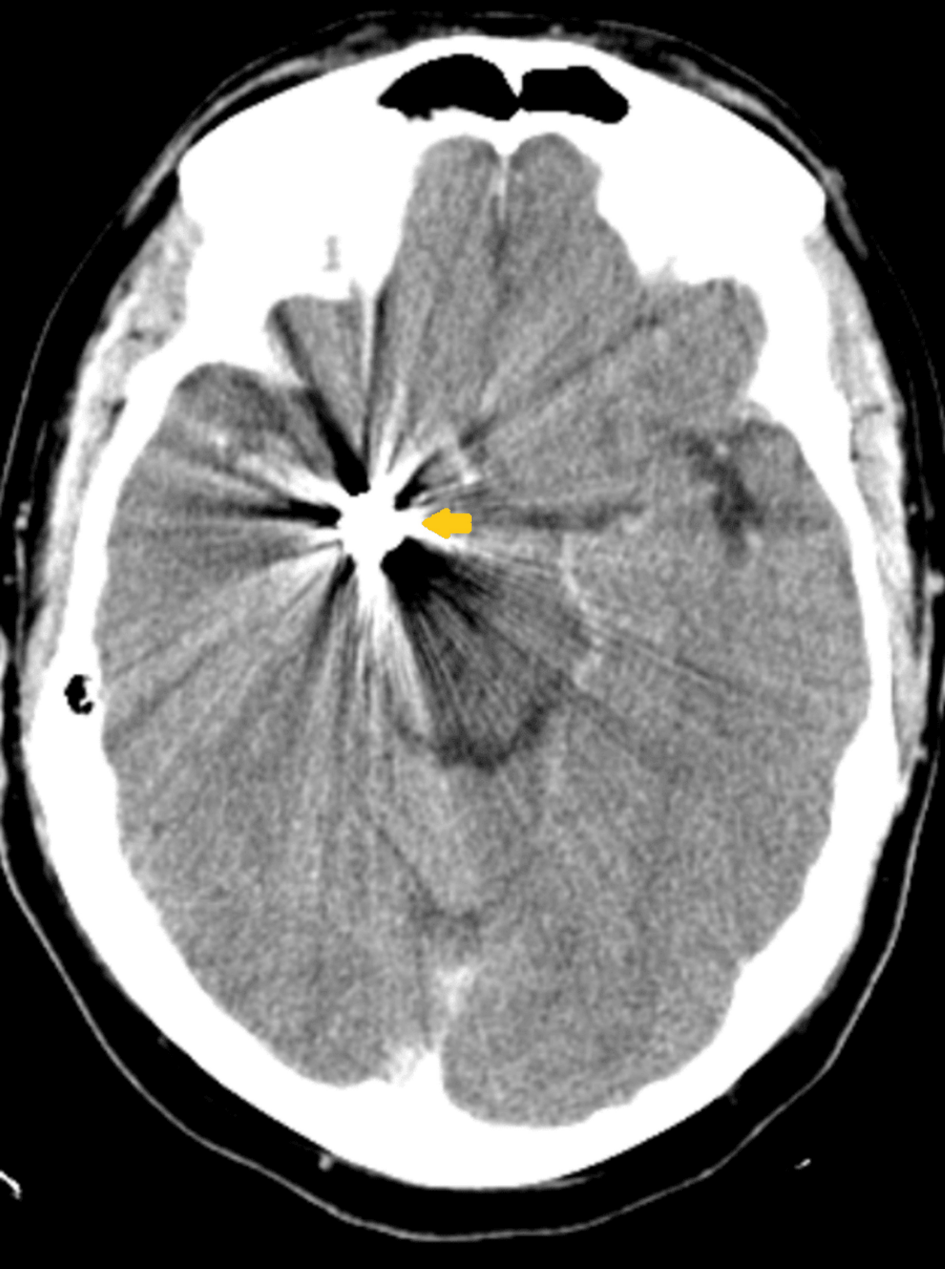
CT of the head showing status post-coil embolization of a right posterior communicating artery aneurysm, with extensive associated metallic streak artifact (yellow arrow), obscuring adjacent structures. No acute territorial infarct. R: right; L: left
